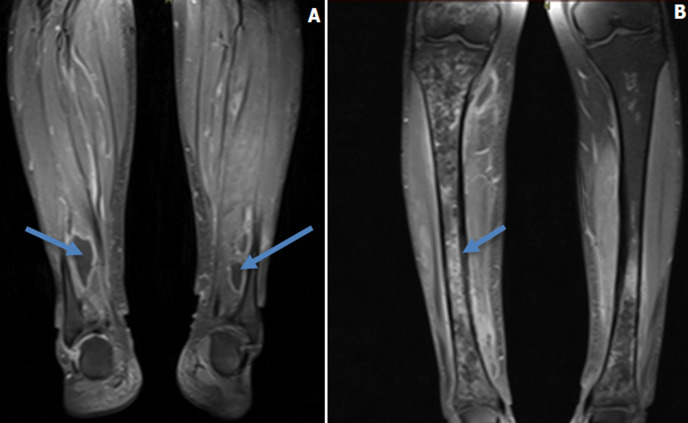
A) post-contrast T1 fat-suppressed MRI image showing bilateral subcutaneous and intramuscular multiple small collections; B) post-contrast T1 fat-suppressed MRI shows subtle enhancement of bone marrow with no cortical break, elevation nor obvious periostitis suggestive of osteomyelitis
